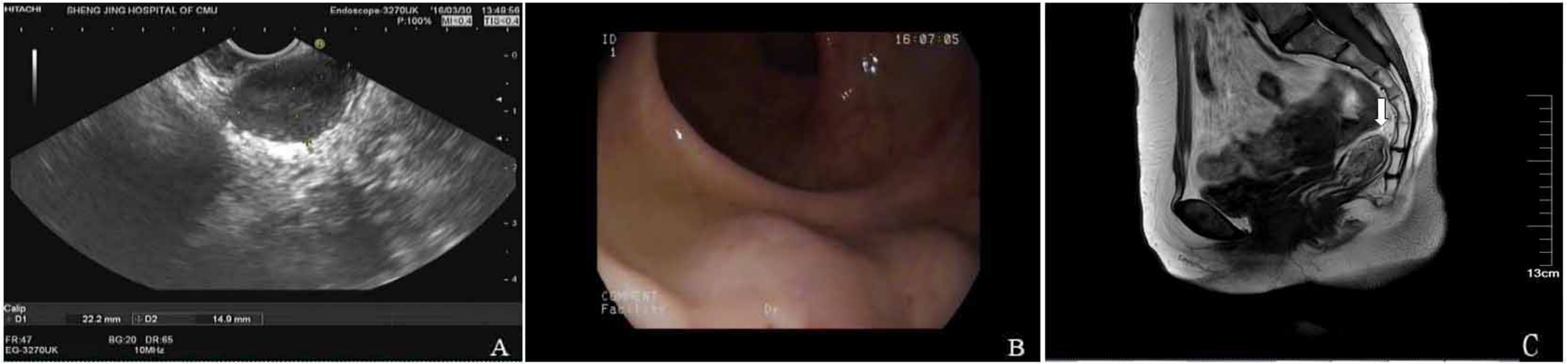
Imaging examination shows the location of the other rectal GIST. a Endoscopic ultrasonography shows that the tumor protruded from the anterior rectal wall. b The colonoscopy results show that the root of the tumor was located only 3 cm above the dentate line. c The results of MR (arrow) showed that the tumor was located in the space of the rectovaginal septum and encapsulated by the rectal muscle, with partially unclear boundary to the vaginal wall
